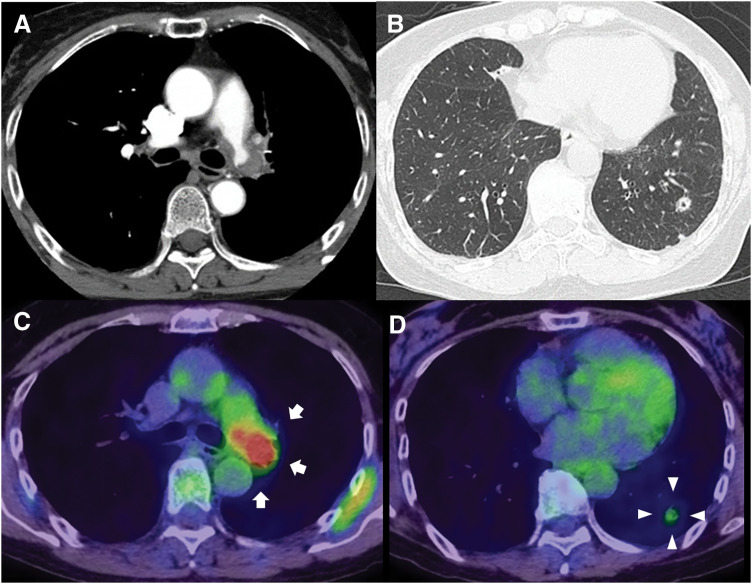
Preoperative imaging findings. Contrast-enhanced chest CT showing a mass encircling and compressing the left main pulmonary artery (A). Chest CT showing a cavitary mass in the left lower lobe using lung window settings (B). PET scan highlighting the left pulmonary artery (arrows) with a maximum standardized uptake value (SUVmax) of 13.3 (C), and a nodule (arrowheads) in the left lower lobe with an SUVmax of 2.0 (D).
